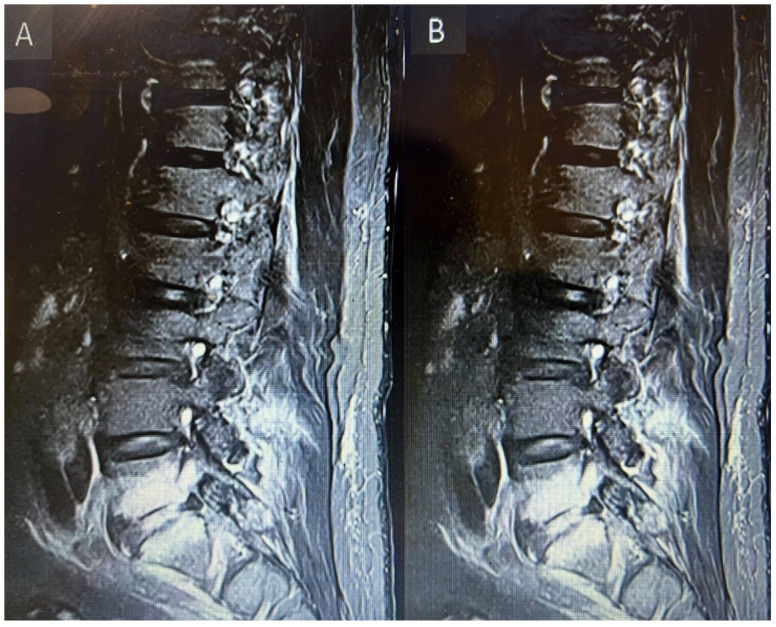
MRI lumbar spine short tau inversion recovery sequency sagittal views of first MRI before starting anti-gout medications (A) and follow-up MRI after starting anti-gout medications (B) showing spondylodiscitis at the level of lumbar 5, sacral 1 level.
